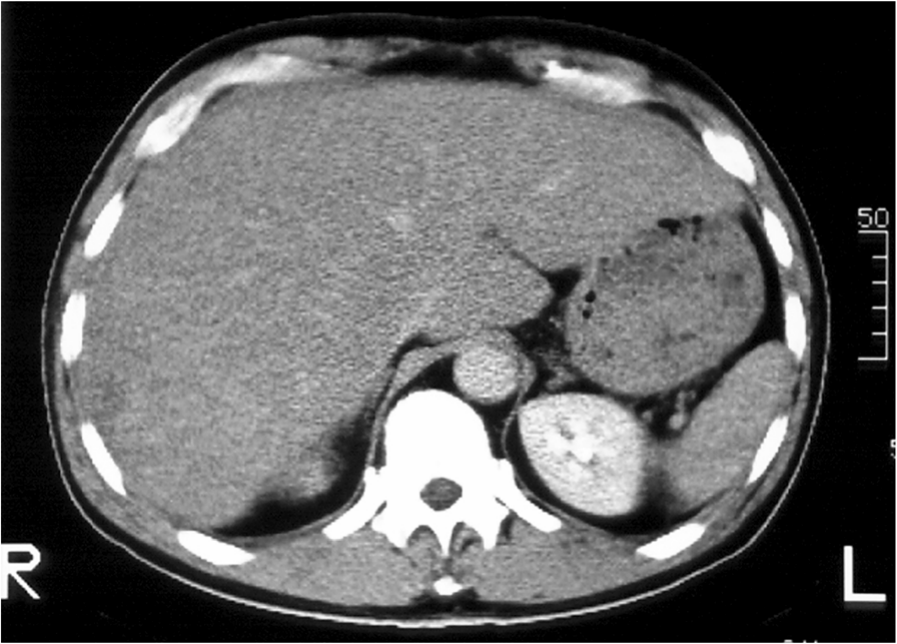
Computed tomography. The liver was remarkably swollen and the parenchyma was diffusely rough
